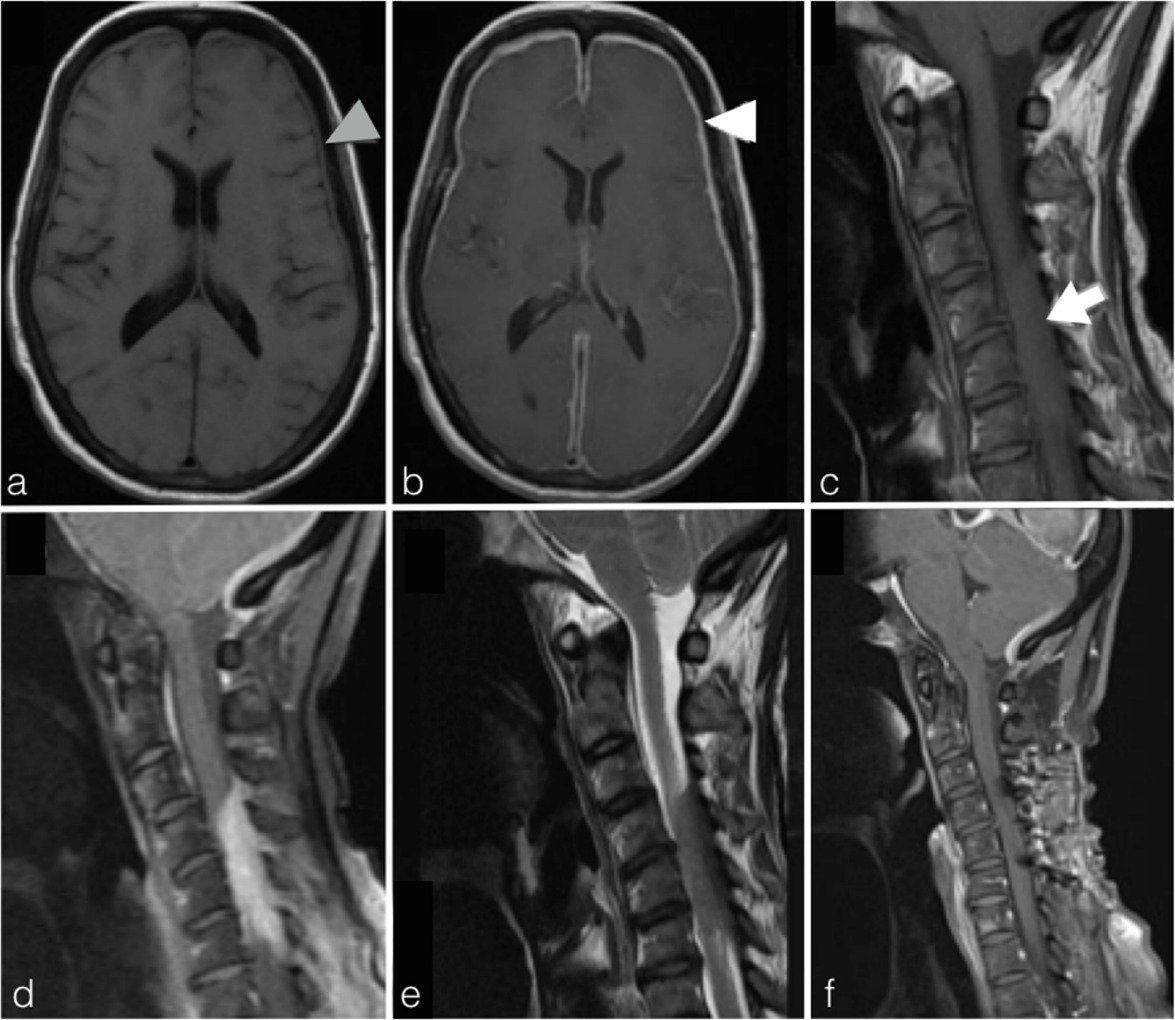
Patient 2. MRI brain scans revealed diffuse bilateral pachymeningeal thickening that was T1-weighted isointense (a, axial, grey arrowhead) and demonstrated intense contrast-enhancement (b, white arrowhead). Cervical spinal imaging also revealed an intradural-extramedullary lesion at the C4/5 level posterior thecal sac that was also T1-weight isointense (c, sagittal, white arrow) and exhibited homogeneous contrast enhancement with a dural-tail sign (d). The spinal lesion caused significant cord compression (T2W, sagittal). After 2 months of prednisolone and azathioprine, a repeat MRI scan showed no recurrent lesion (f, contrast-enhanced T1W)
